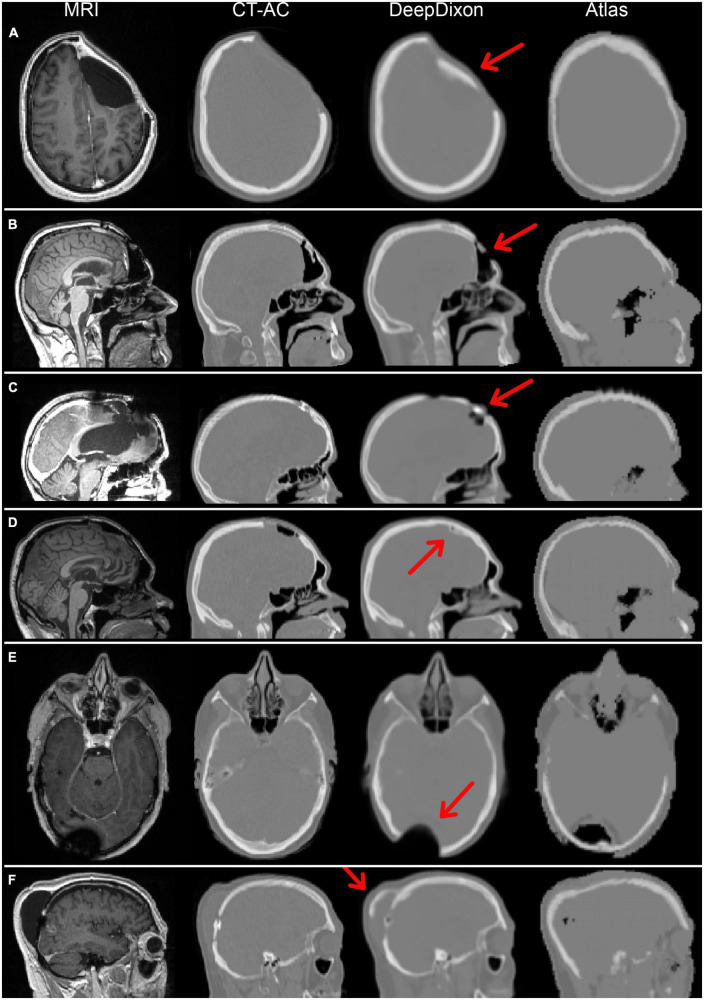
Magnetic resonance imaging (MRI) and attenuation maps for all six patients with DeepDixon artifacts categorized as major. Two patients had follow-up scans, both with consistent artifacts, resulting in a total of 8/318 scans with major artifacts. The patients in (A–C,E) had biological tumor volume (BTV) > 1 ml, and were therefore included in the positron emission tomography (PET) evaluation. The primary errors were caused by false bone formation along titanium implant/soft tissue (A,D,F), or directly on brain in postoperative pneumocephalus (B), and metal associated signal voids (C,E).
